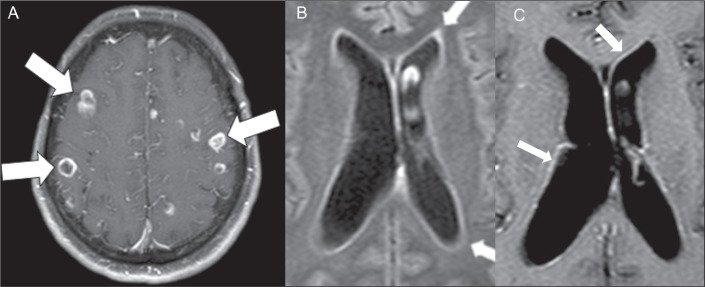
MRI scans of two different patients. A: Cryptococcomas.
